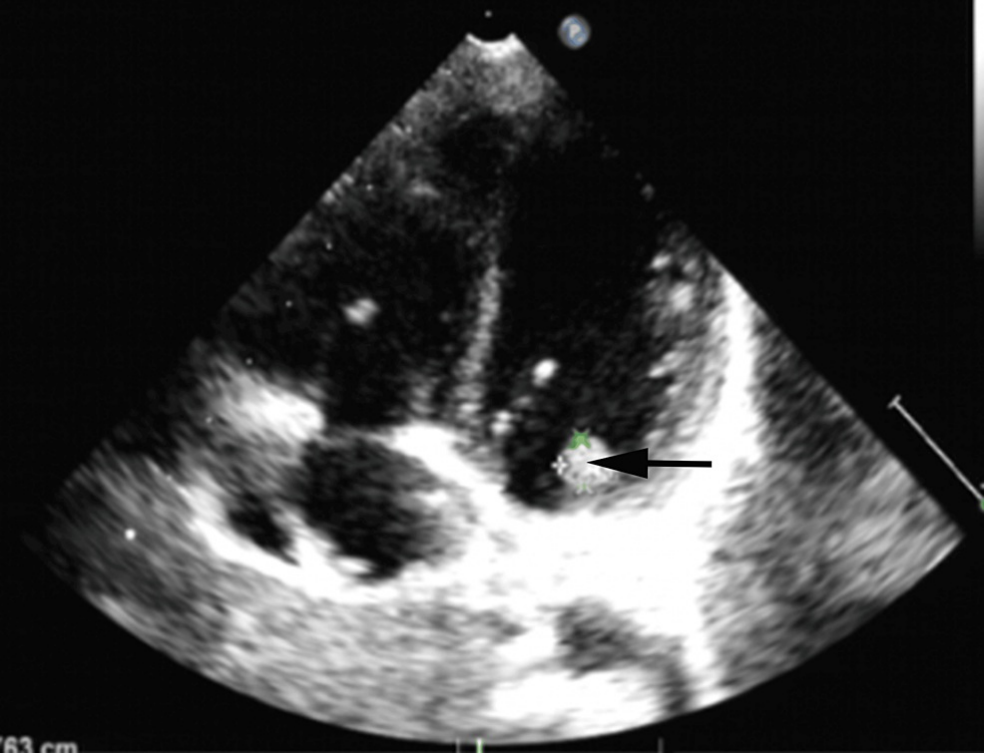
Echocardiography demonstrates hypoechoic mitral valve cusp (black arrow)
